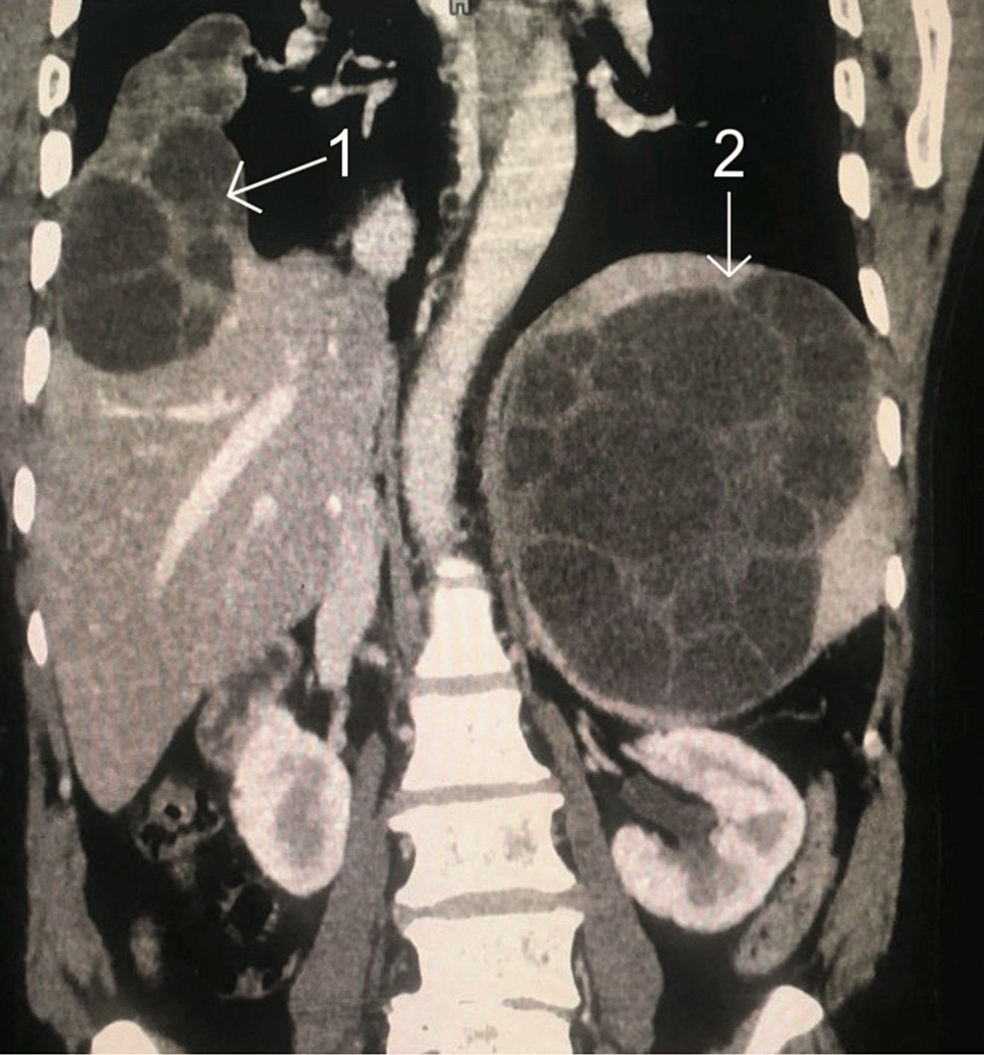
Computed tomography image showing a view of hydatid cysts presence in the liver and spleen. 1, hydatid cyst in the liver; 2, hydatid cyst in the spleen
